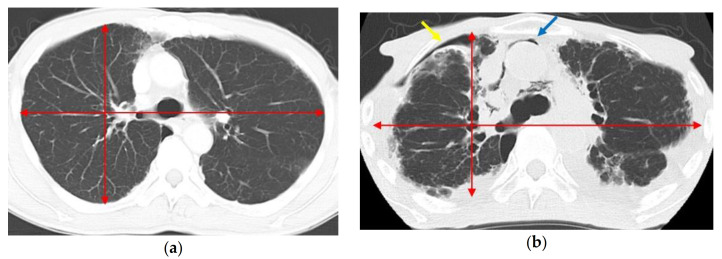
Chest CT images of a patient with idiopathic pleuroparenchymal fibroelastosis (IPPFE). (a) Chest CT before the diagnosis of IPPFE at the level of the sixth thoracic vertebra. The bilateral lungs are almost normal. (b) Chest CT 7 years later at the same level. The anteroposterior diameter of the thoracic cage has become shortened. Shrinking of the lungs has resulted in an air space in the right chest cavity (yellow arrow) and pneumomediastinum (blue arrow), indicating air leak syndrome. Abbreviations: CT—computed tomography; IPPFE—idiopathic pleuroparenchymal fibroelastosis.
